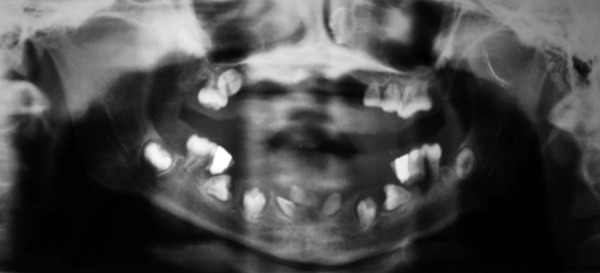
Orthopantomogram shows status of dentition affected by DD-I after 3 years
